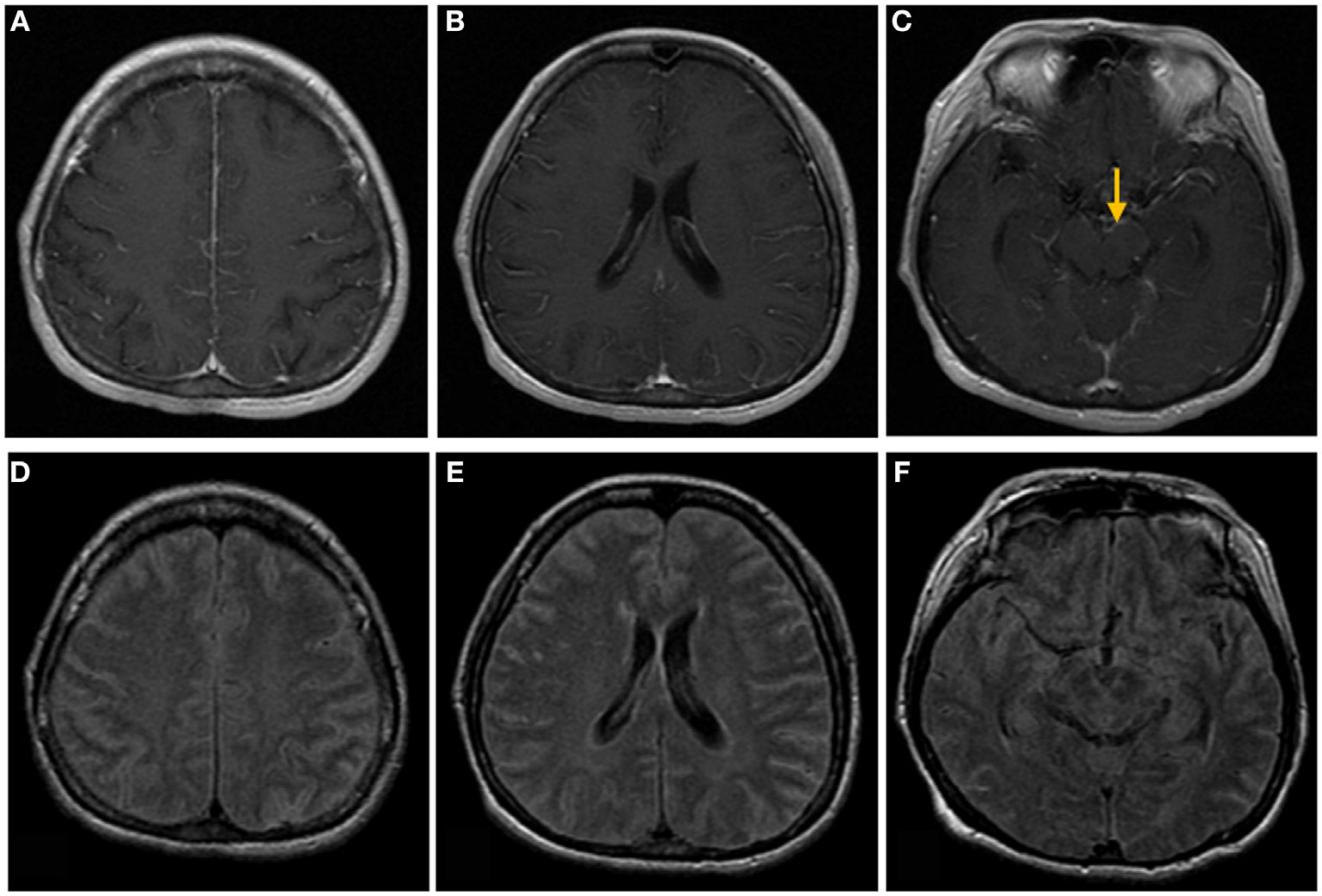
Brain magnetic resonance images of a 50-year-old woman with headache, nausea, and vomiting. (A–C) Post-contrast T1-weighted images show linear meningeal enhancement along the cerebral sulci and midbrain surface (arrow). (D–F) Fluid-attenuated inversion recovery images show high signal intensities occupying the subarachnoid space, compatible with tuberculosis meningitis.
